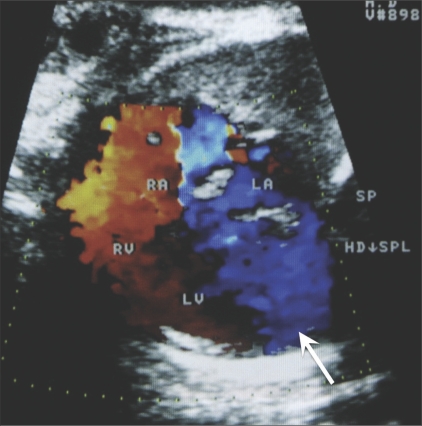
Color Doppler imaging shows massive tricuspid regurgitation and the dyskinetic left ventricle and aneurysm (arrow) (RA - right atrium, RV - right ventricle, LA - left atrium, LV - left ventricle)
